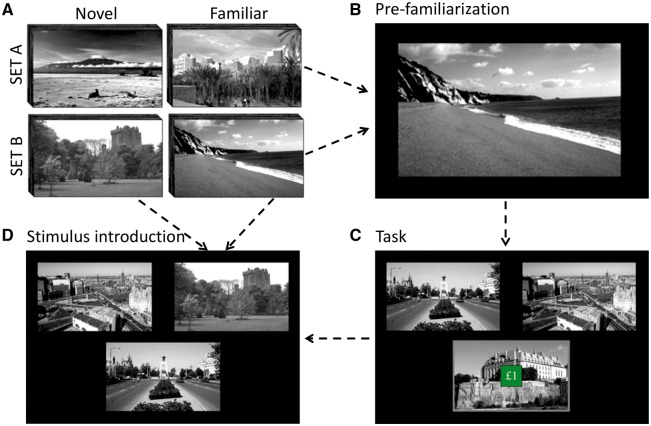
Novelty processing task. (A) Image sets: A set of 64 greyscale pictures (SET A or SET B) was randomly allocated for each session. (B) Pre-familiarization: participants were familiarized to half of the image set by passive, then active viewing. (C) Three-armed bandit task: during functional MRI, participants chose between three options (images) on each trial. Each option had a fixed probability (mean: 33%) of winning £1. Participants were instructed to choose options that maximized their total reward. Each trial consisted of stimulus presentation (3.5 s), choice feedback (3 s), and reward feedback (superimposed £1 or £0) (1.5 s). If participants failed to respond, ‘No response’ was displayed (4.5 s). There was a jittered intertrial interval (1.5–3 s). Option locations were randomly shuffled between trials. (D) On 25% of trials one option was randomly replaced by a new one from the image set. Images differed in reward value, but novel and familiar (pre-familiarized) images had the same reward probability distributions (mean 33%). The task was split into three 13-min runs, each containing 80 consecutive trials.
